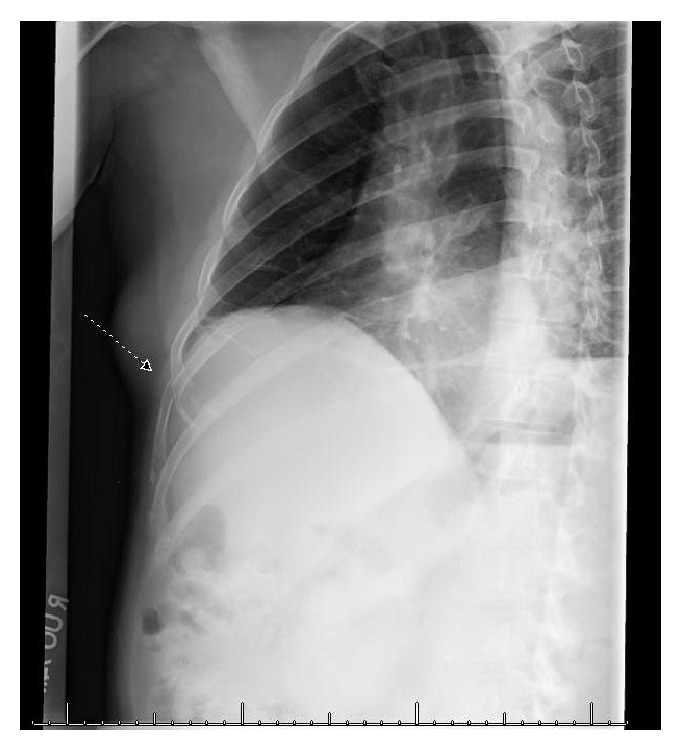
X-ray of the right sided ribs revealing a fracture involving the lateral aspect of the 7th rib.
